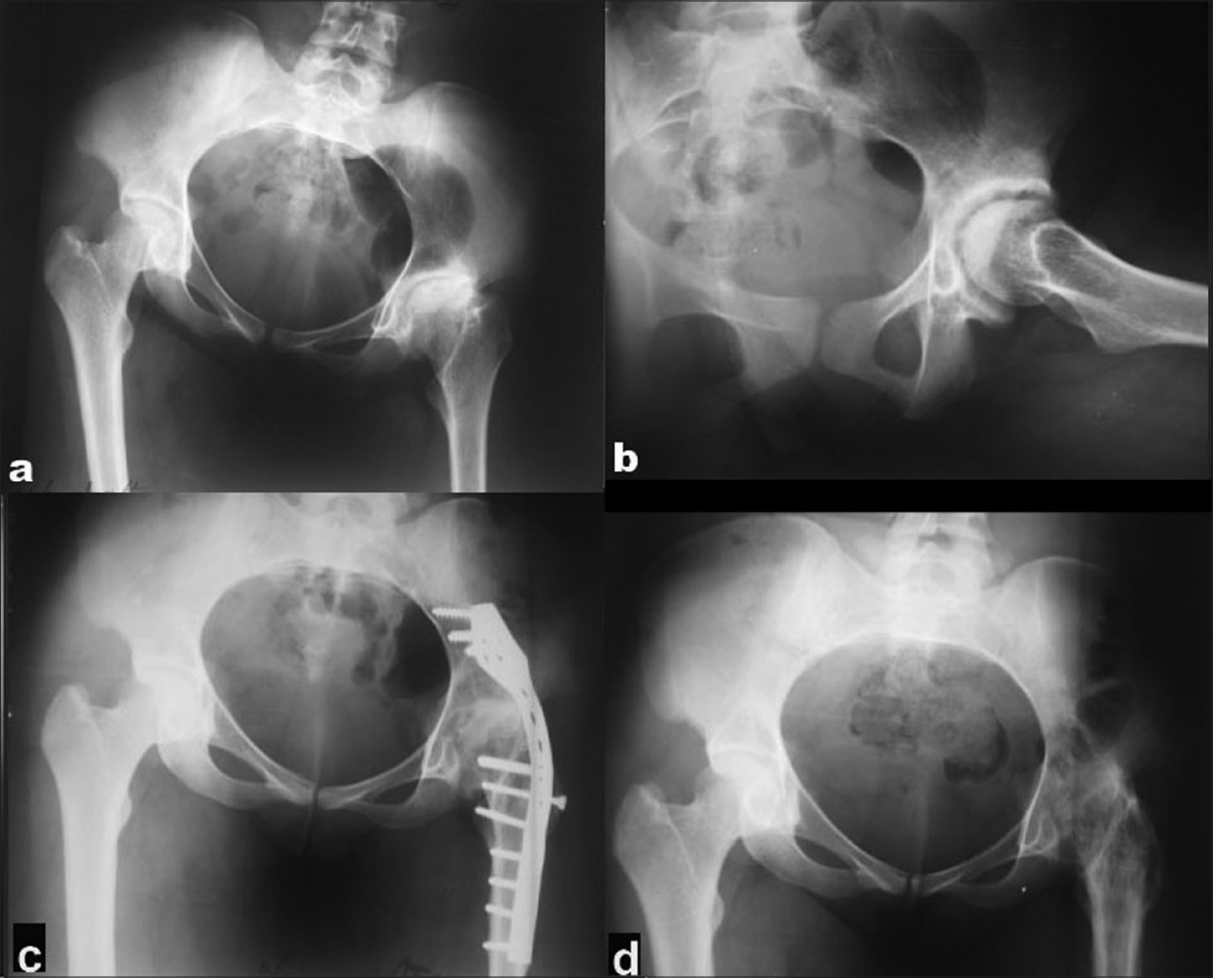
(a) Pre-operative X-ray of pelvis including both hips (anteroposterior view) and lateral view of left hip (b) showing post-infective hip with reduced joint space, coxa breva, and subchondral sclerosis on both femoral and acetabular sides. (c) X-ray of pelvis including both hips (anteroposterior view) showing Cobra plate fixation of the left hip with obliteration of the joint space. (d) Removal of implant showing trabecular bone growth across the joint at 10.5 years follow-up
